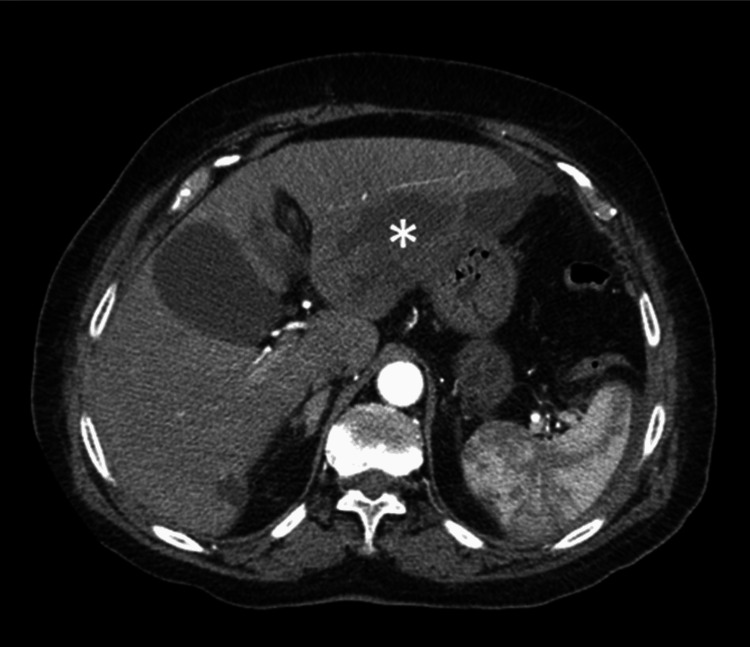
Abdominal computed tomography scan showing a large multiloculated abscess in the left hepatic lobe (asterisk)
